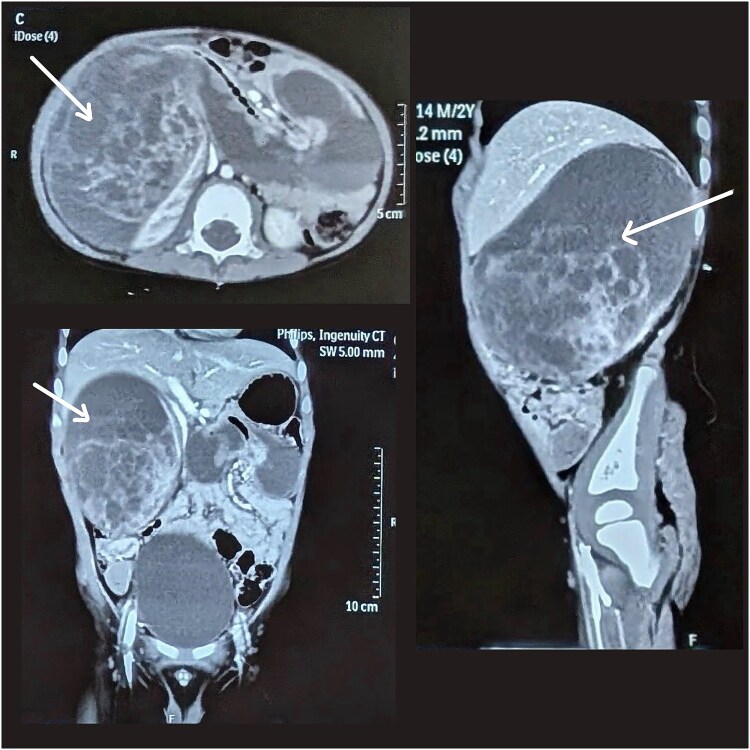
Axial, coronal, and sagittal sections of a CT scan showing a large heterogeneously hypodense space-occupying lesion in the right kidney, suggestive of Wilm tumor of the right kidney.
